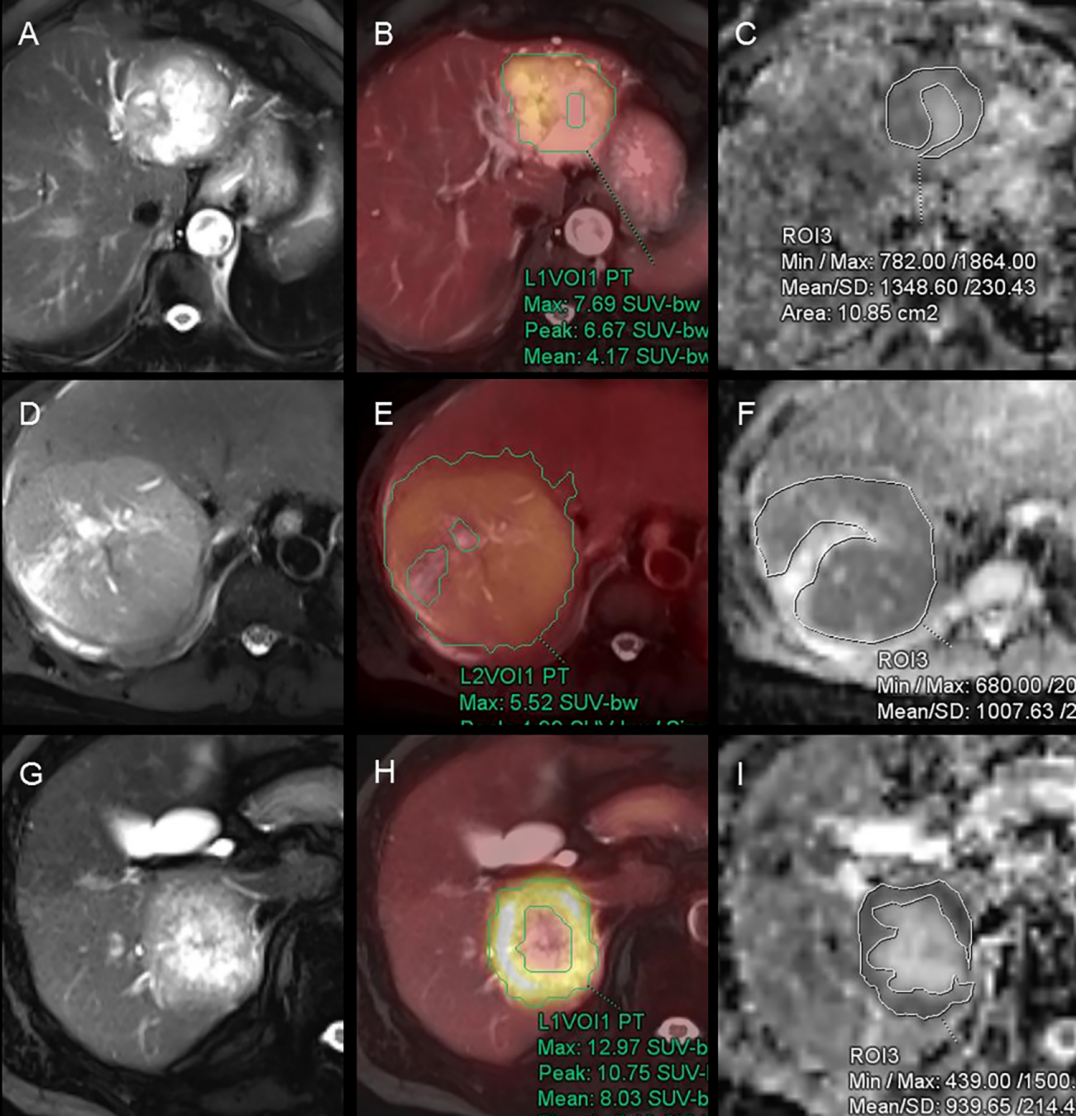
F-18 fluorodeoxyglucose (FDG) positron emission tomography (PET)/ magnetic resonance (MR) images with region of interest (ROI). (A–C) FDG PET/MR images of 85-year-old woman with cholangiocarcinoma; (D–F) 46-year-old man with hepatocellularcarcinoma; (G–I) 72-year-old man with hepatic metastasis from colon cancer. (A, D and G) Axial HASTE MRI images; (B, E and H) Fused images of FDG PET and HASTE; (C, F and I) ADC map. ROIs were manually drawn along the contour of the tumor except necrosis. HASTE, half-Fourier acquisition single-shot turbo spin-echo; ADC, apparent diffusion coefficient.
